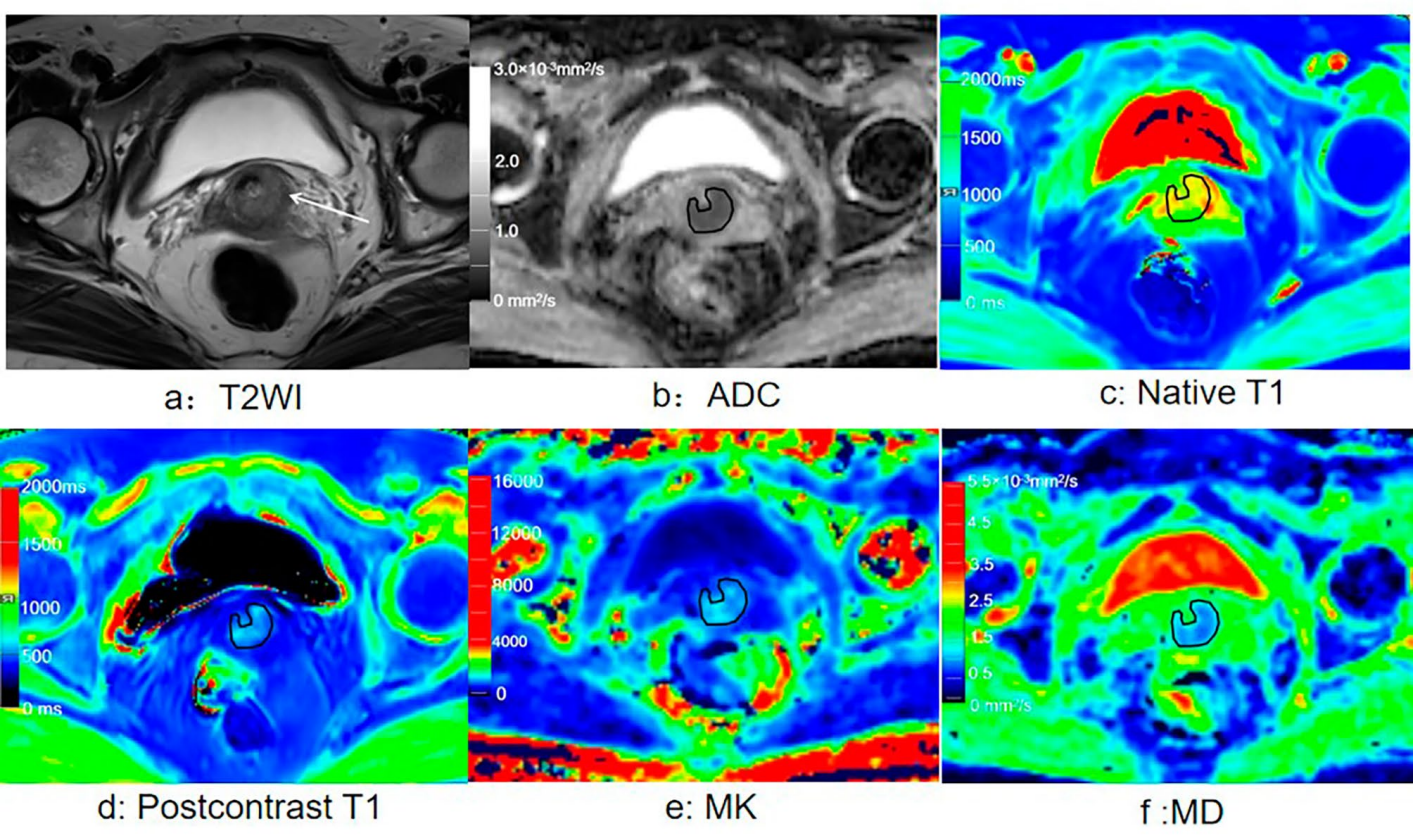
cervical squamous cell carcinoma (stage IB, high-grade). (a) Axial T2-weighted image shows a mass with a slight high-intensity signal in the cervix. (b) The mass showed low intensity on the ADC map. ADC value = 0.68 × 10 − 3 mm2/s. C) Native T1 map. d) Postcontrast T1 map. e) MK map. f) MD map
